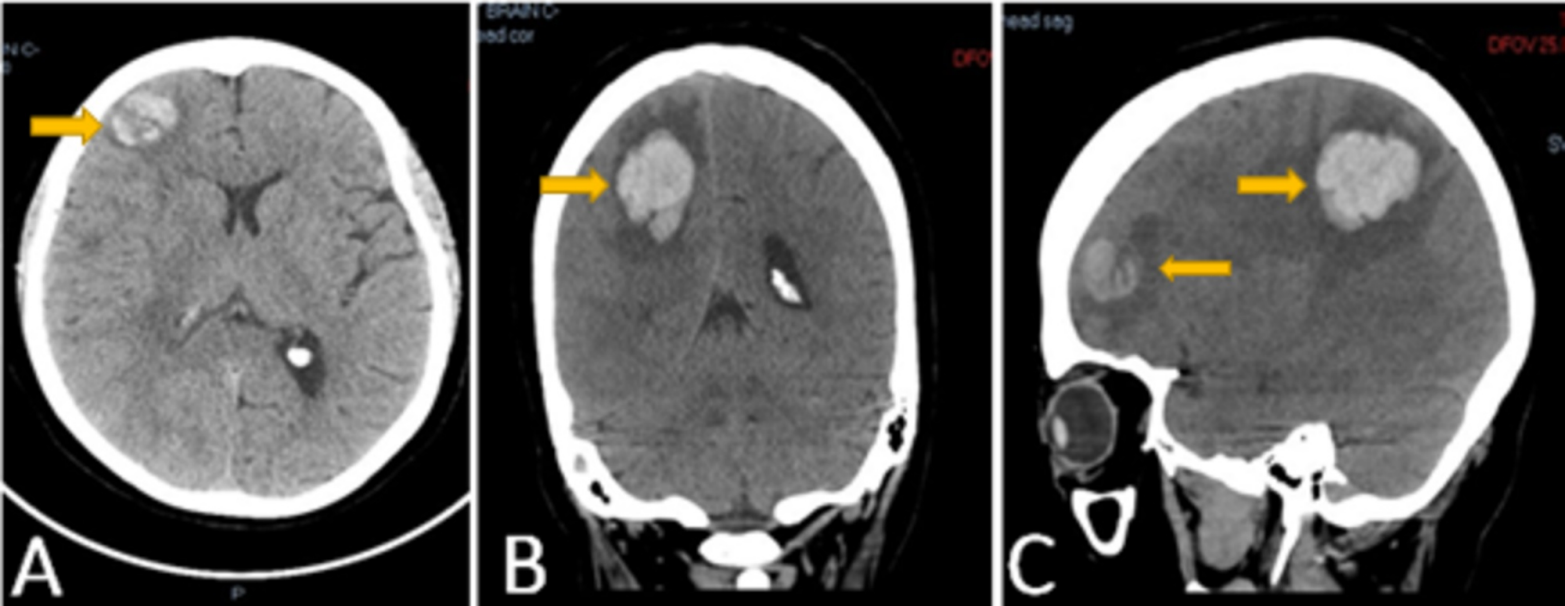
Computed tomography (CT) of the brain Figure A (axial) and B (coronal) views represent a 2.2 cm right frontal lobe area of intraparenchymal hemorrhage with surrounding edema and mild mass effect. Figure C shows a sagittal view, revealing a right parietal lobe, large, 3.7 x 2.5 cm, hyperdense; and a smaller area within the right frontal lobe with surrounding vasogenic edema and possible related underlying mass.
